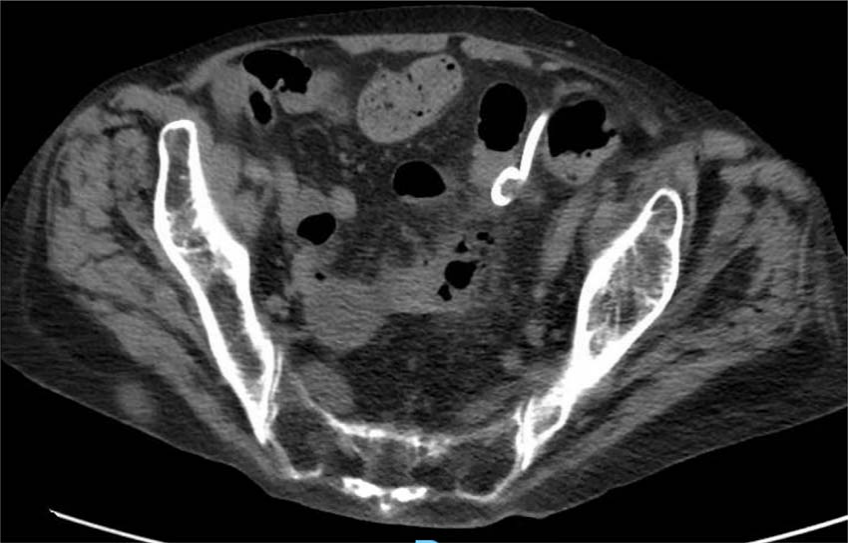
CT showing resolution of abscess with IR pigtail drain in place.
